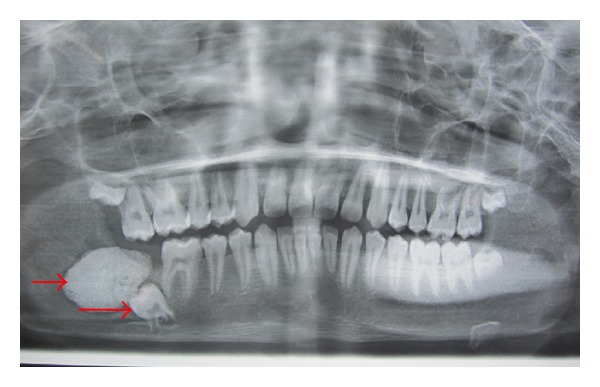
Orthopantomograph revealing radioopaque lesion in the right mandibular angle region involving the impacted second molar.
